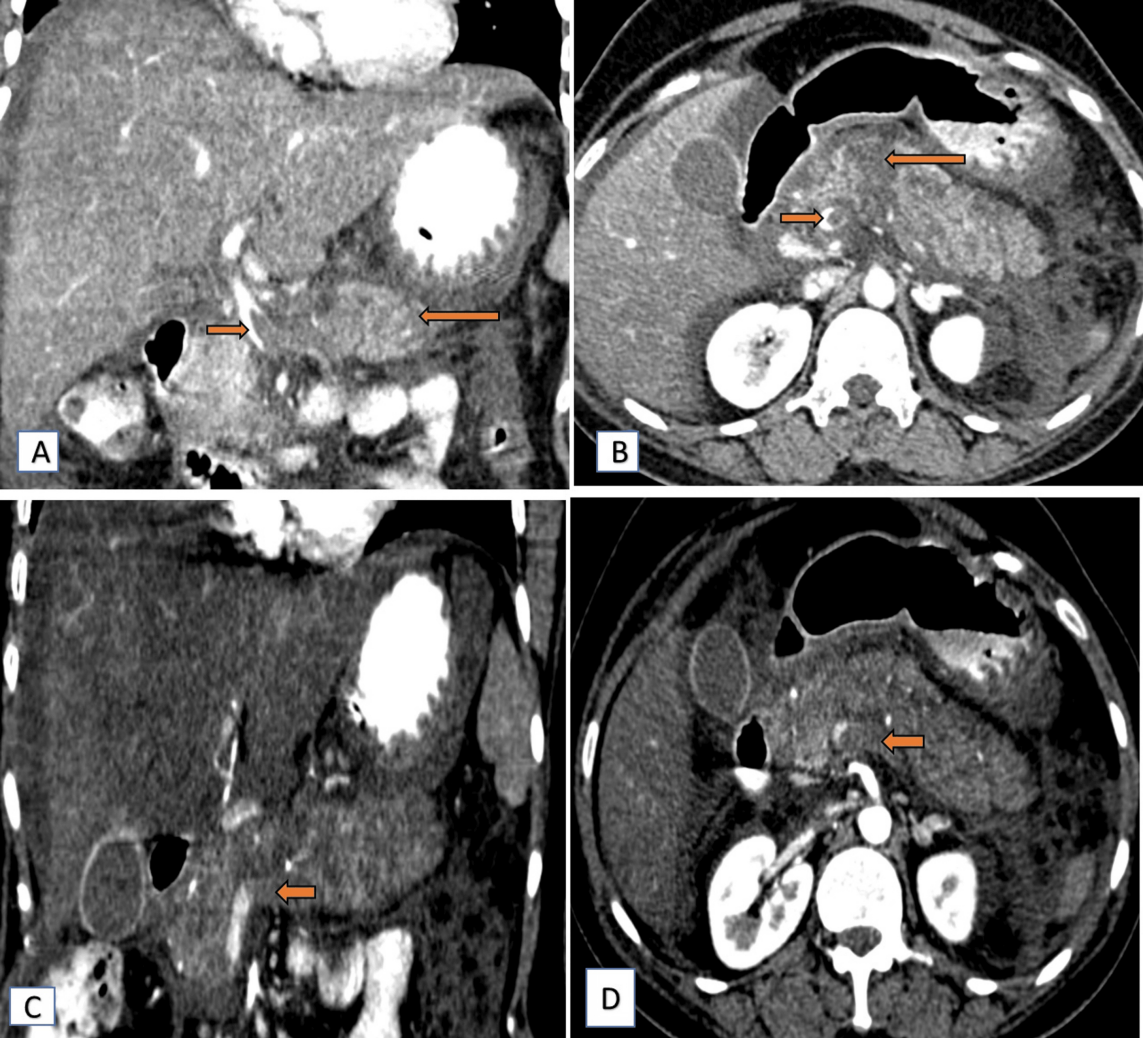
Contrast CT images (coronal and axial) showing thrombosis in the portal vein and splenic vein Contrast CT images (portal phase) reveal a hypodense filling defect in the portal vein at the region of the portal vein confluence in coronal (short arrow) (A) and axial (short arrow) (B) sections. It also shows heterogeneous pancreatic parenchymal attenuation with a few small, hypodense, non-enhancing areas in coronal (long arrow) (A) and axial (long arrow) (B) sections, suggestive of necrotic areas. Contrast CT images (arterial phase) reveal a complete non-opacification of the splenic vein in coronal (short arrow) (C) and axial (short arrow) (D) sections. CT: computed tomography
